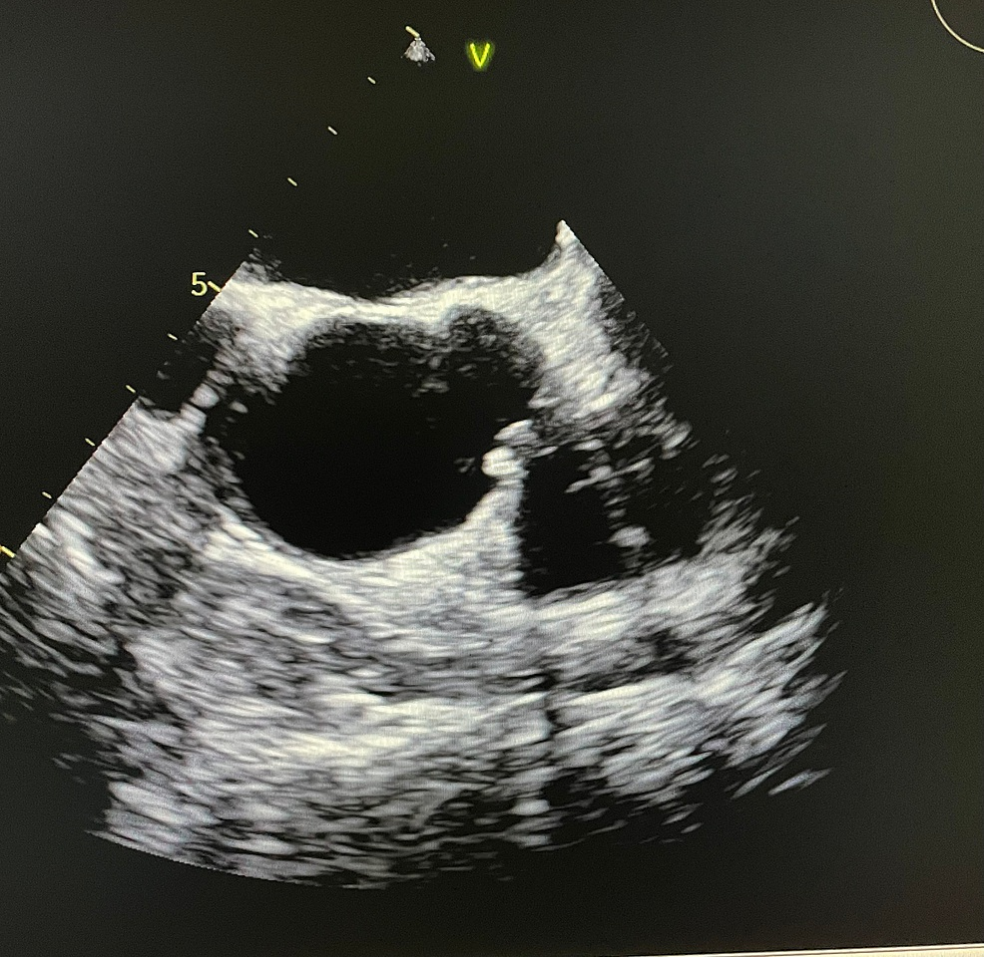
TEE on the following morning did not demonstrate a cardiac mass TEE: Transesophageal echocardiography
